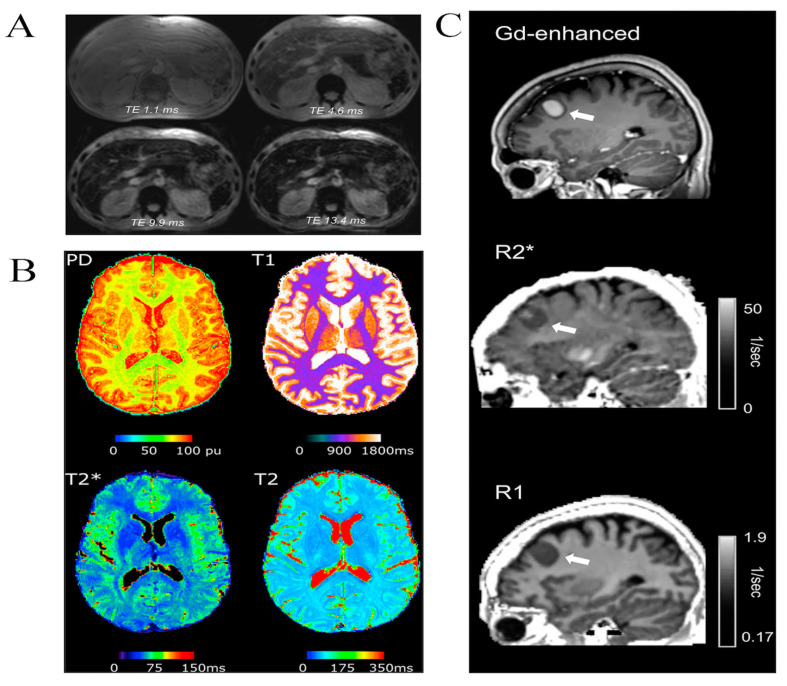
(A) Gradient-echo images of the liver at echo times of 1.1, 4.6, 9.9, and 13.4 ms. Reprinted with permission from [57], Copyright ©2008, Informa Healthcare USA, Inc. (B) Examples of quantitative MRI maps of a single subject. PD: Proton density. Reprinted with permission from Ref. [58], Copyright © 2019 Elsevier Inc. (C) From top to bottom: Gd-enhanced T1-weighted image, R1 map, and R2* map in a representative subject with a meningioma brain tumor (white arrow); reproduced with permission from Yu E et al. [59], under a Creative Commons Attribution 4.0 International License (http://creativecommons.org/licenses/by/4.0/, accessed on 21 March 2024).
